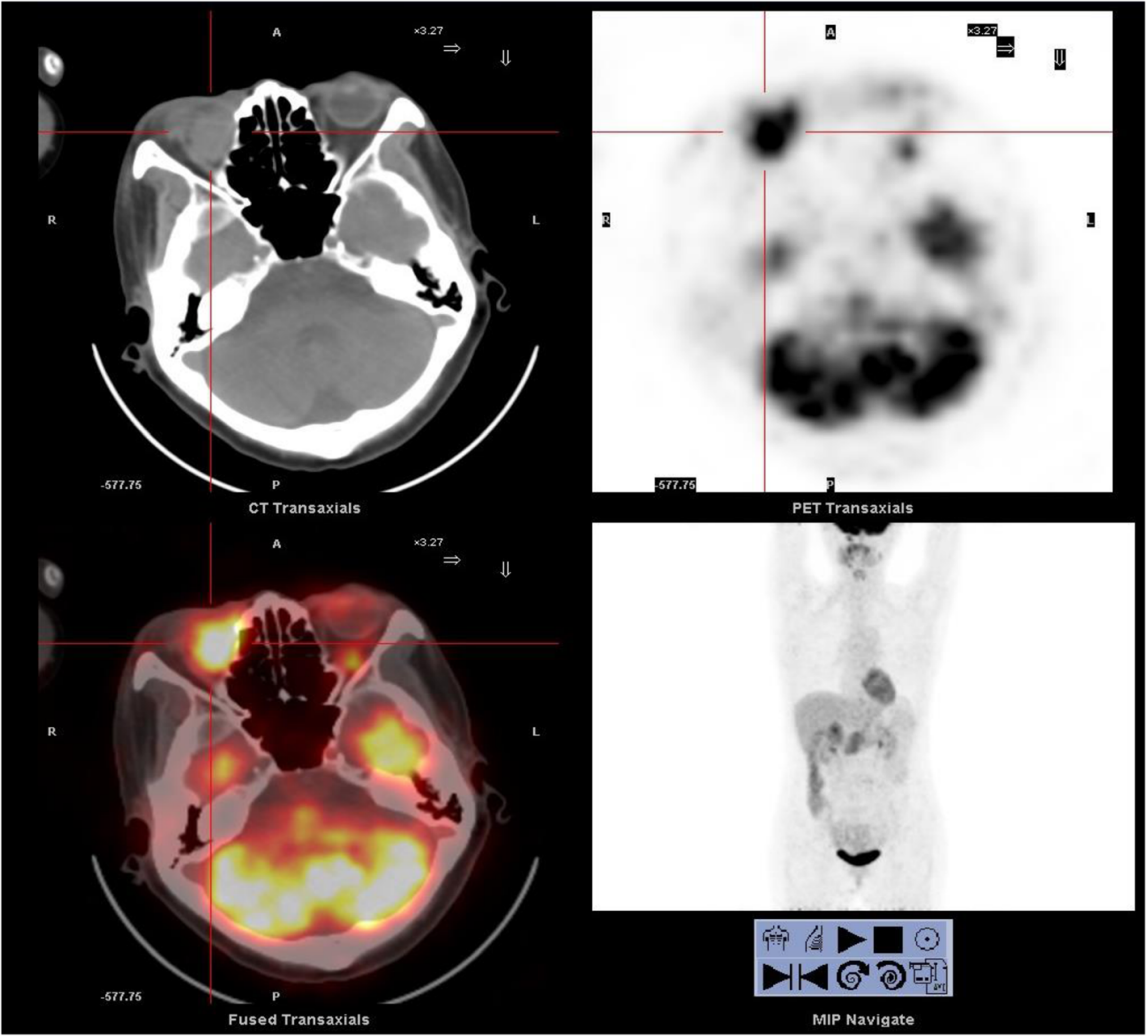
A systemic ^18^F-2-Fluoro-2-Deoxy-D-Glucopyranose positron emission tomography (^18^F-FDG PET/CT) demonstrated an orbital mass with increased FDG uptake but no other remarkable malignancy in the trunk
